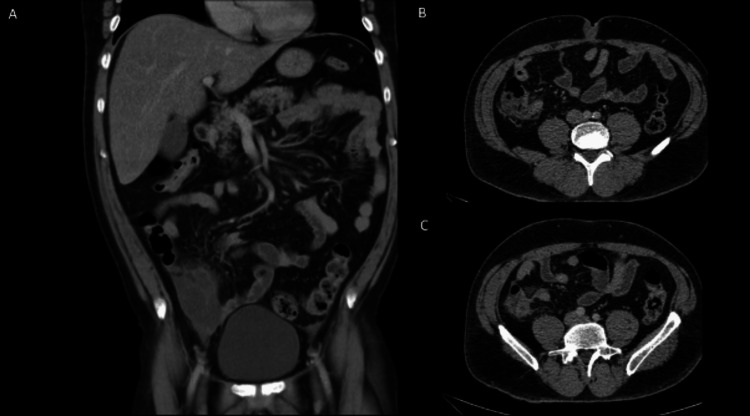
Computed tomography of the abdomen and pelvis with contrast (Case 4). (A) Coronal section; (B) axial section demonstrating a 7 cm diameter fluid collection in the right iliac fossa, lateral and inferior to the cecum. Centrally within this fluid collection, there was a low-grade, tubular, grossly thick-walled structure suggestive of a large appendiceal abscess.
